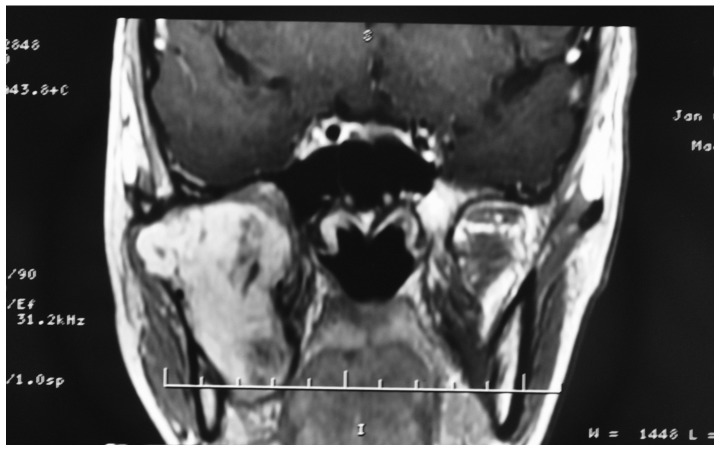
Coronal magnetic resonance imaging (MRI) scan showing a well-defined 5.1×3.6-cm mass within the right infratemporal fossa (ITF).
